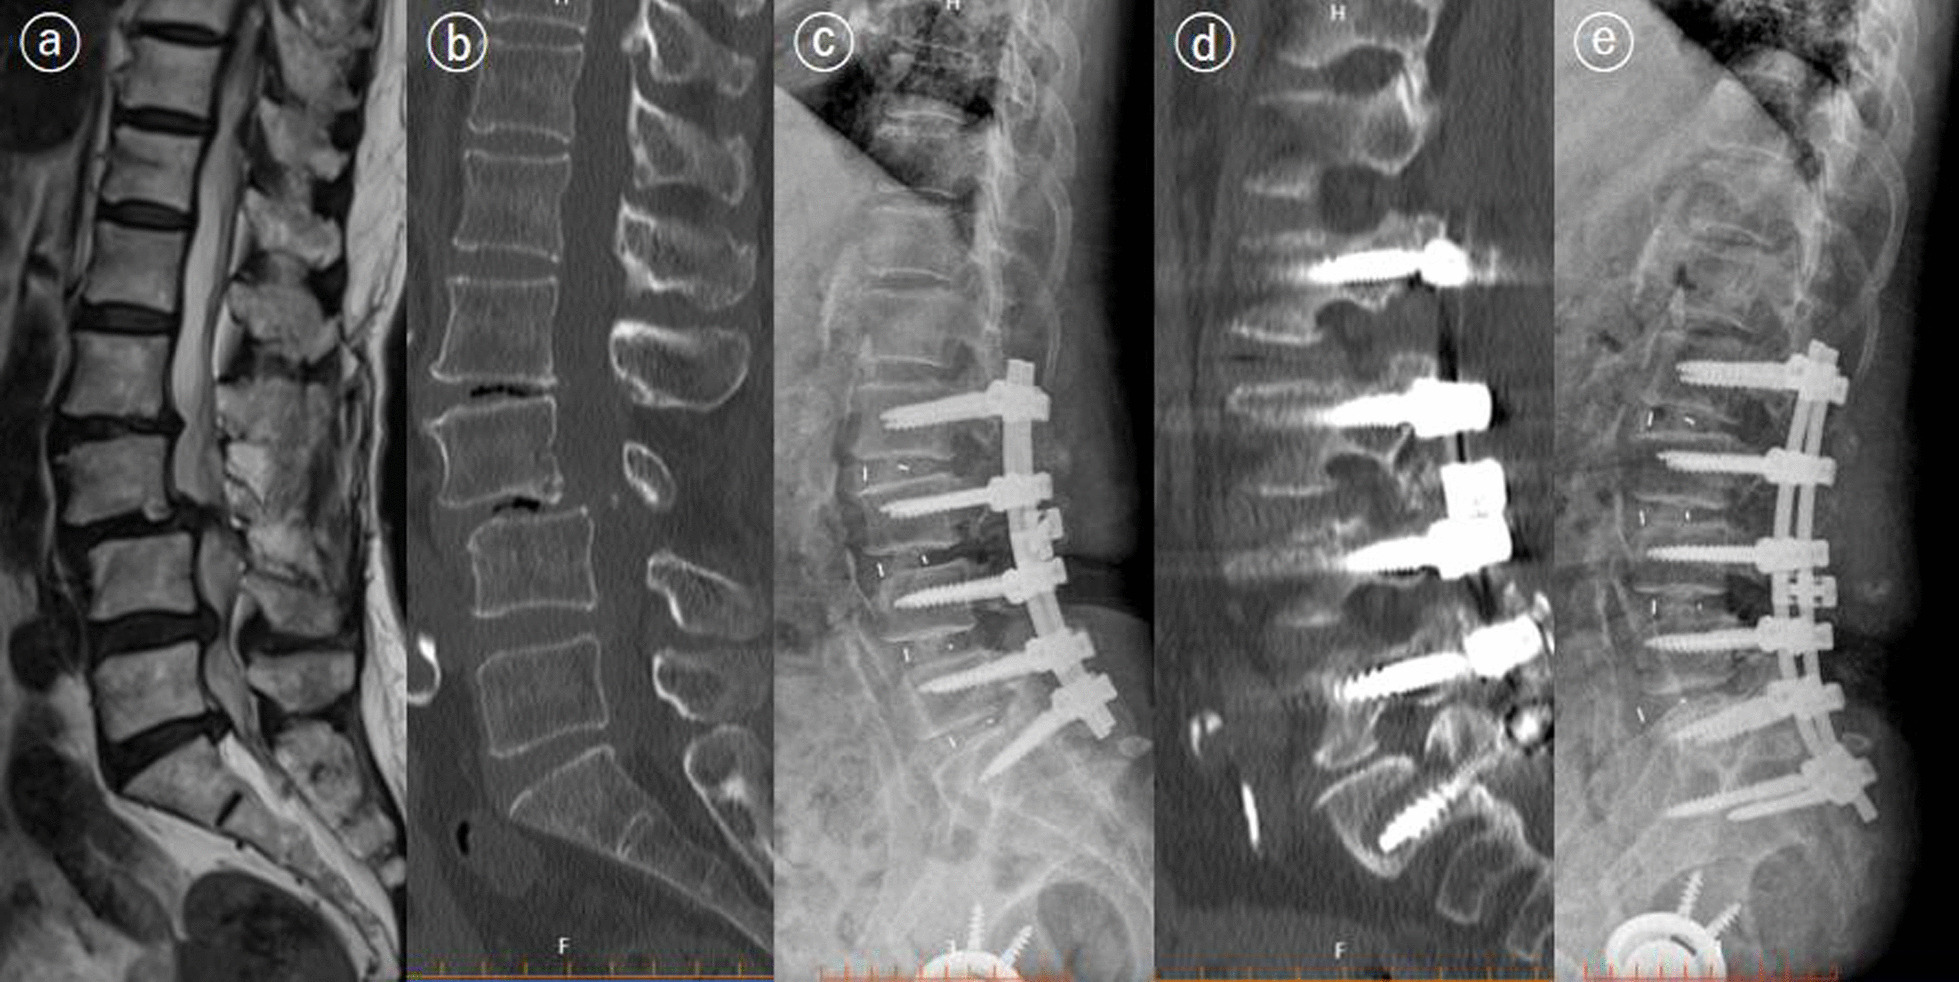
A 74-year-old patient underwent second surgery for screw misplacement. The patient underwent posterior decompression and fusion surgery for L3–L4 grade 2 spondylolisthesis and stenosis (L4–L5, L5–S1) (a, b). The postoperative X-ray was normal (c). The patient had moderate pain, and CT revealed bone union and screw displacement at the 1-year follow-up (d). The patient underwent revision surgery and achieved adequate pain control (e)
